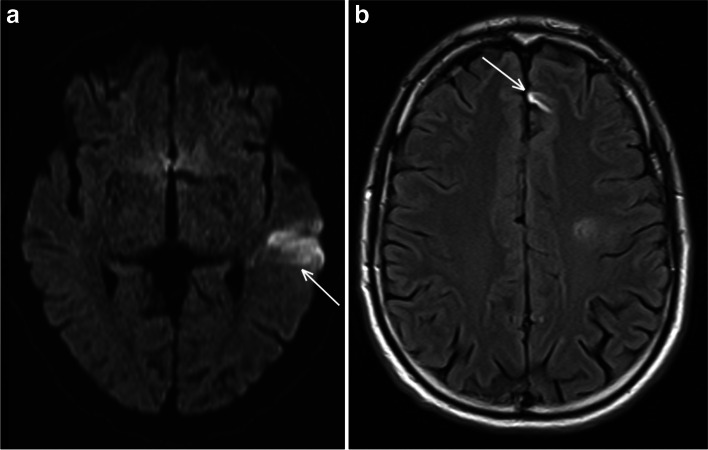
Occurrence of two new lesions compared to in the previous MRI. A left temporal lobe hypersignal on axial DWI MRI related to a recent ischemic lesion in the left MCA territory (a) and an older one in the ACA territory, only visible on axial FLAIR sequences (b)
